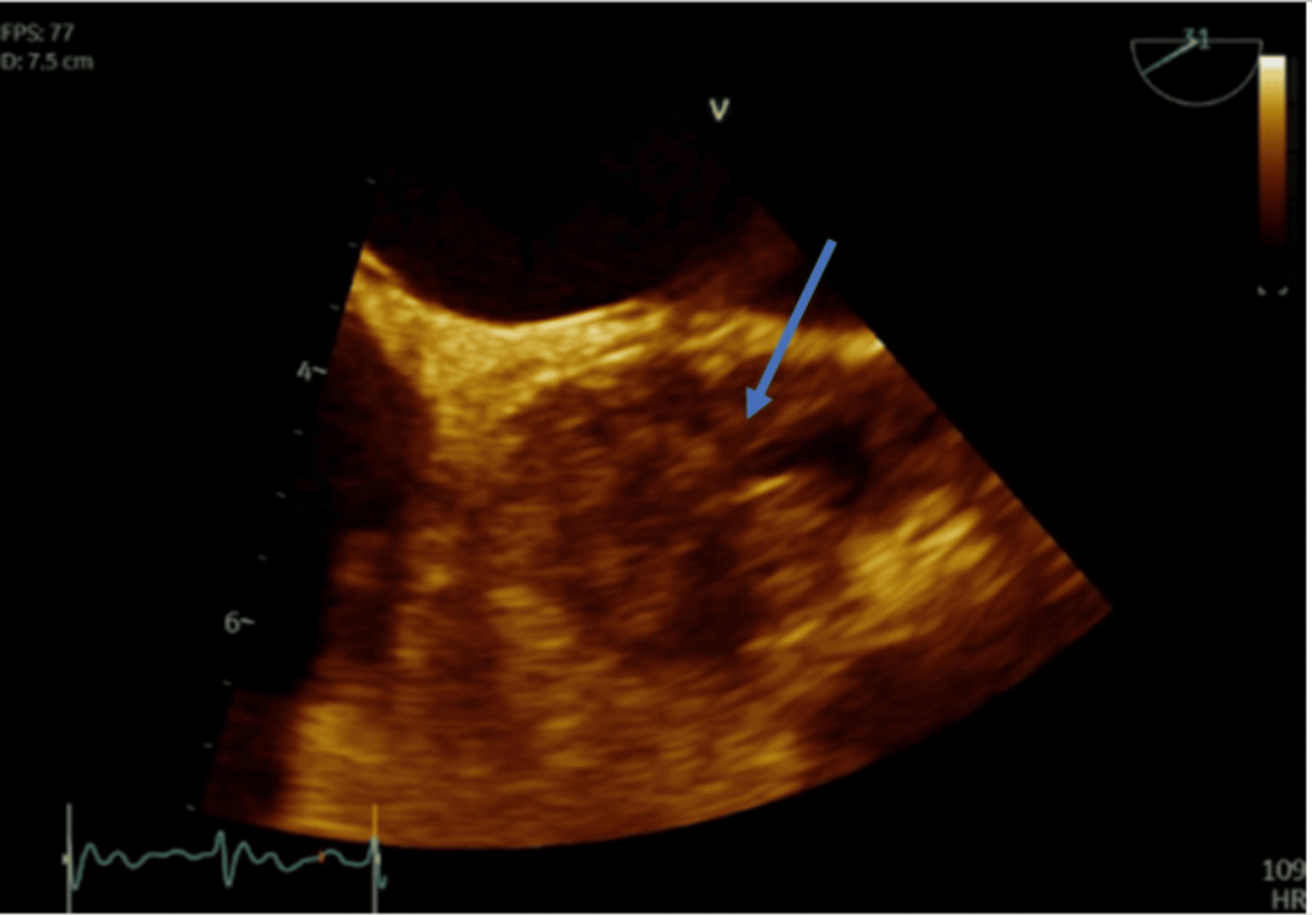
TEE, mid-esophageal short-axis view showing thrombus (arrow) of the AV along all three coronary cusps extending into the LMCA ostium TEE: Transesophageal echocardiogram; AV: Aortic valve; LMCA: Left main coronary artery
